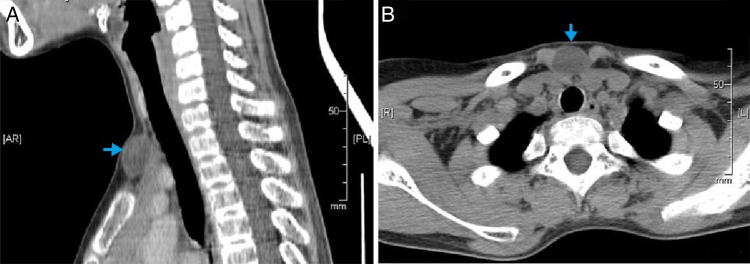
(A) CT neck sagittal showing the cyst (blue arrow) in the suprasternal area. (B) Axial contrasted CT scan of neck showing the cyst and its relation to the surrounding structure.
